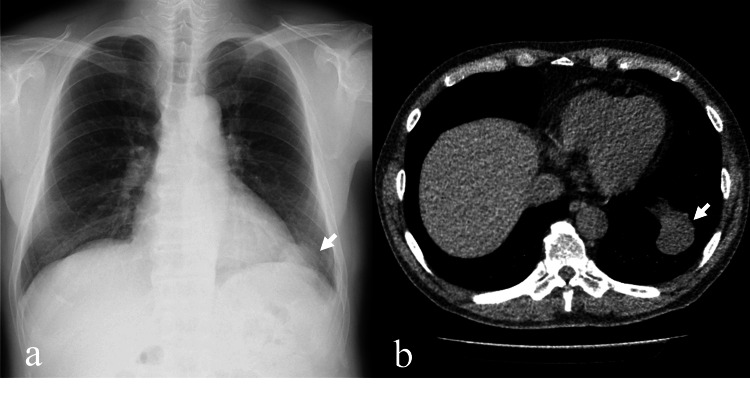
Chest X-ray and CT images at initial diagnosis (a) The chest X-ray revealed a nodular shadow in the left lower lung field (arrow). (b) The chest CT showed a 3.7 cm mass with clear boundaries in the left lower lobe, directly adjacent to the diaphragm (arrow).
